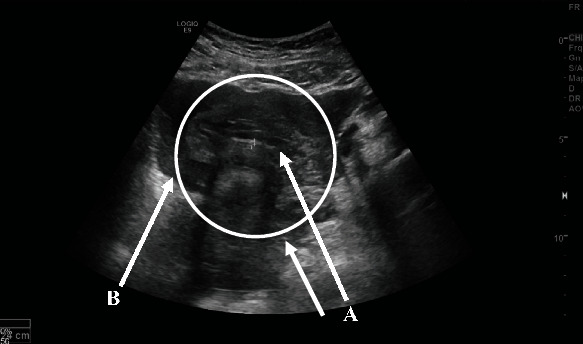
Pelvic sonography showing an empty uterus with thin endometrium (A) and free fluid (B).
